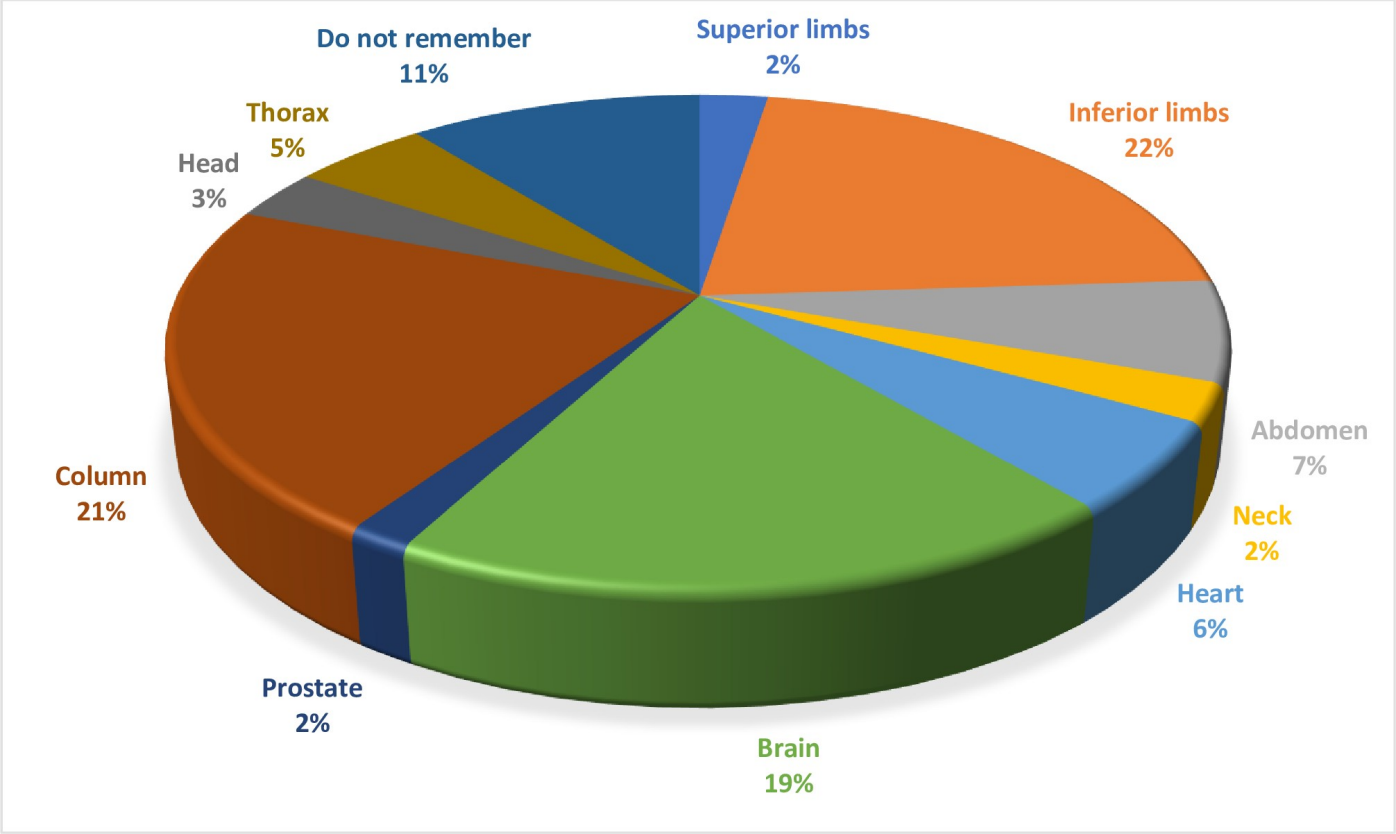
Sites of MRI scan request.
